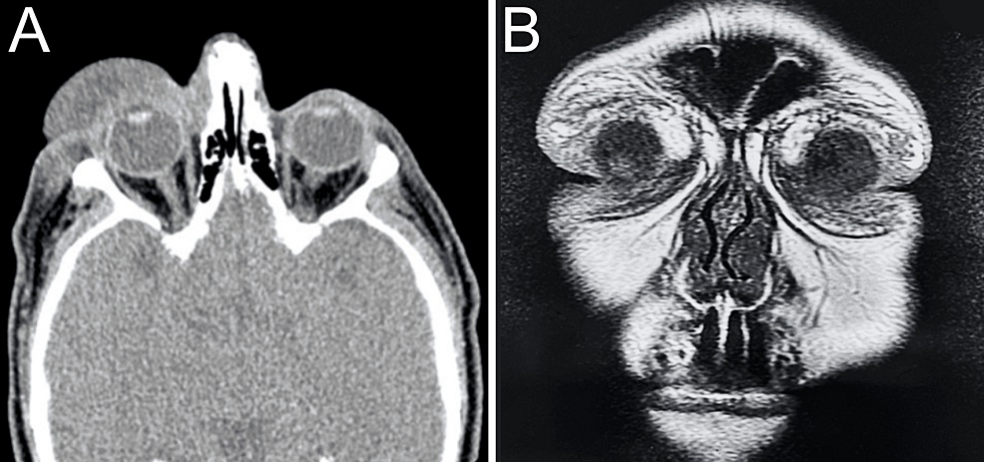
Orbital computed tomography and magnetic resonance imaging of the patient. (A) Orbital computed tomography reveals that the preseptal space was completely infiltrated by the lesion. (B) Magnetic resonance imaging confirms the infiltration of the postseptal spaces within both orbits.
